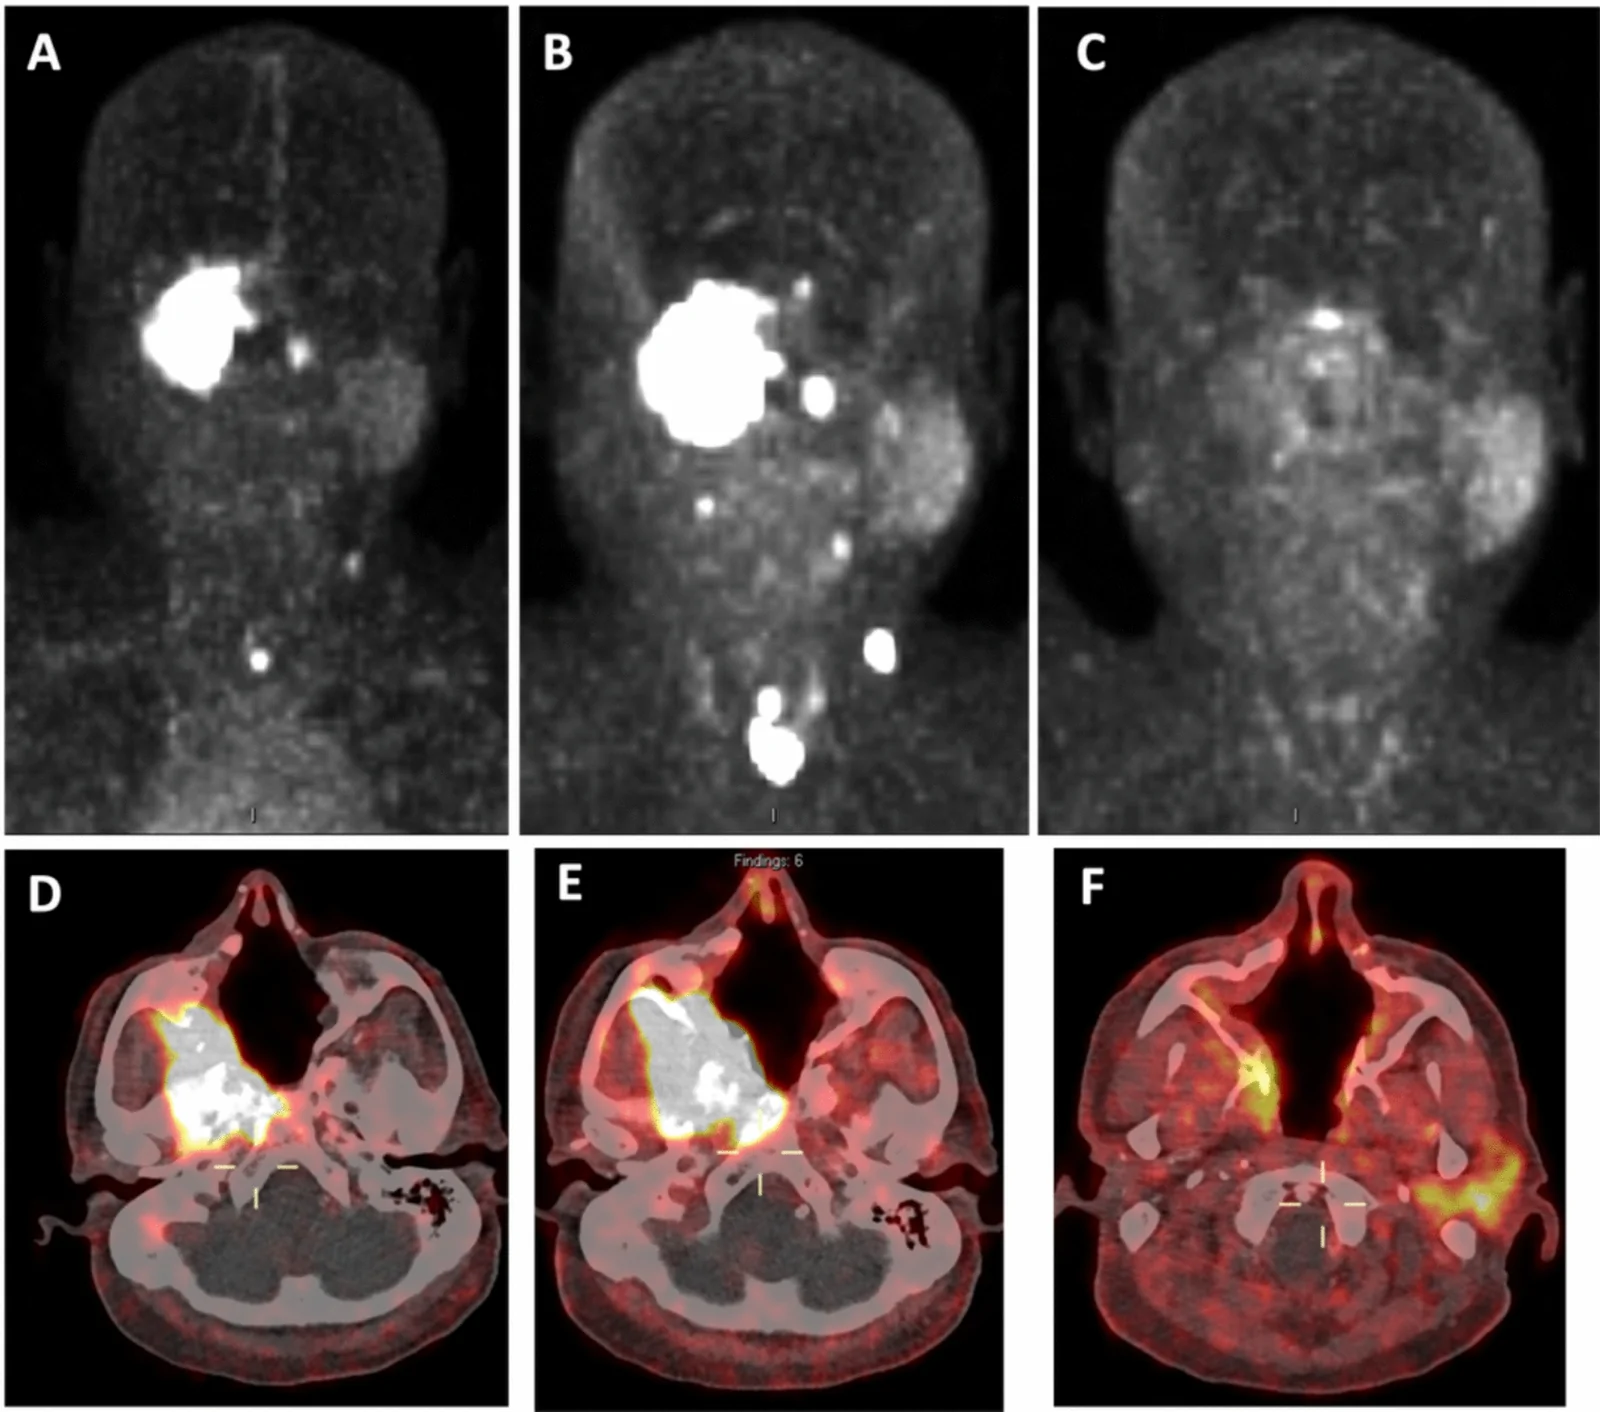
Sequential ^68^Ga-DOTATATE PET/CT imaging of patient with prolonged SD by RECIST 1.1 and metabolic response. A-C: Coronal PET images; D-F: Axial PET/CT images through plane of local recurrence. A,D: baseline imaging showing uptake in local recurrence and cervical lymph nodes; B,E: first restaging at week 9 of treatment showing increased uptake in both primary and cervical disease sites; and C, F: subsequent restaging at week 26 of treatment showing resolution of uptake
